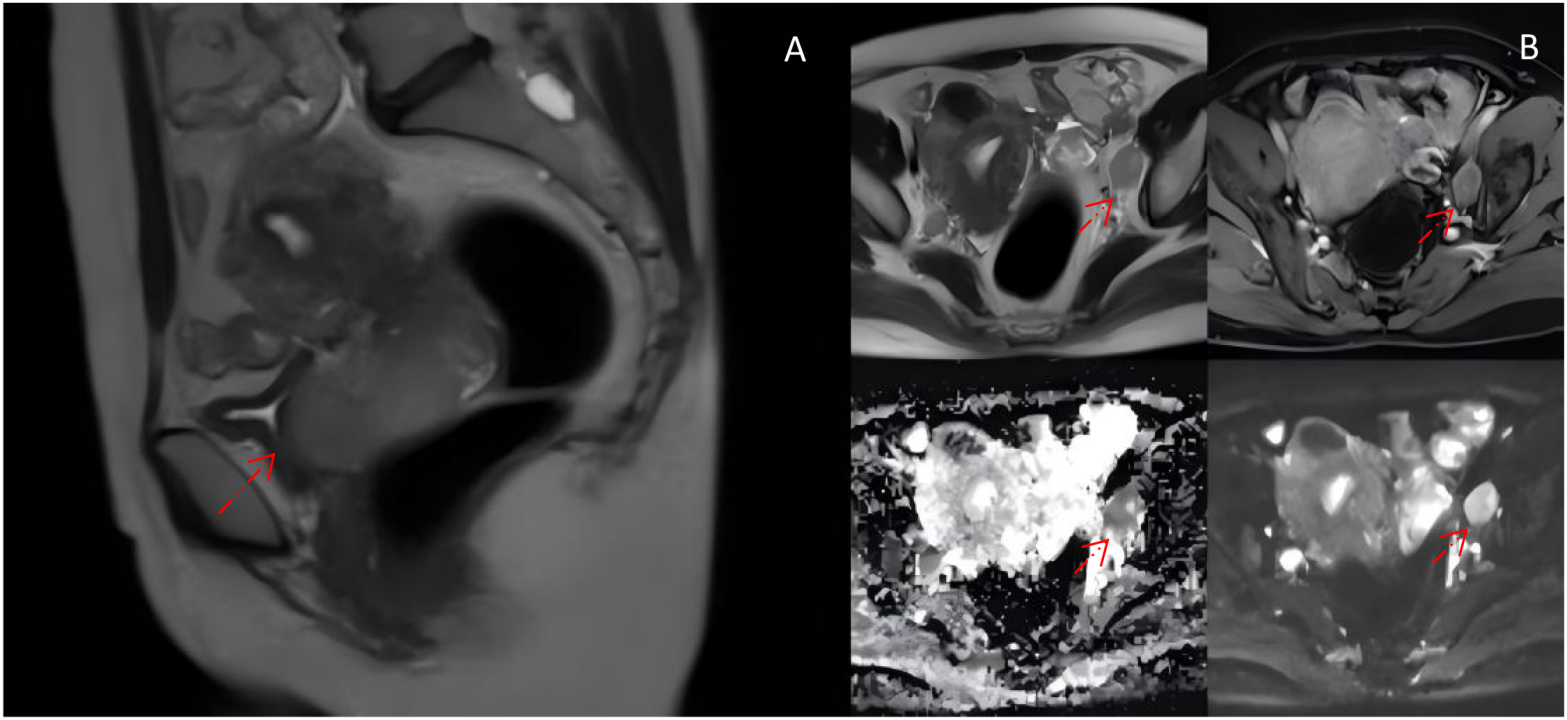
(A) T2 magnetic resonance imaging with contrast of the pelvis showing a lesion in the cervix 5.4 × 6.1 (AP diameter) × 6.6 (craniocaudal). (B) Initial axial diagnostic MRI showing a metastatic lymph node (arrow) 3.0 × 1.8 (AP diameter) × 3.1 (craniocaudal).
